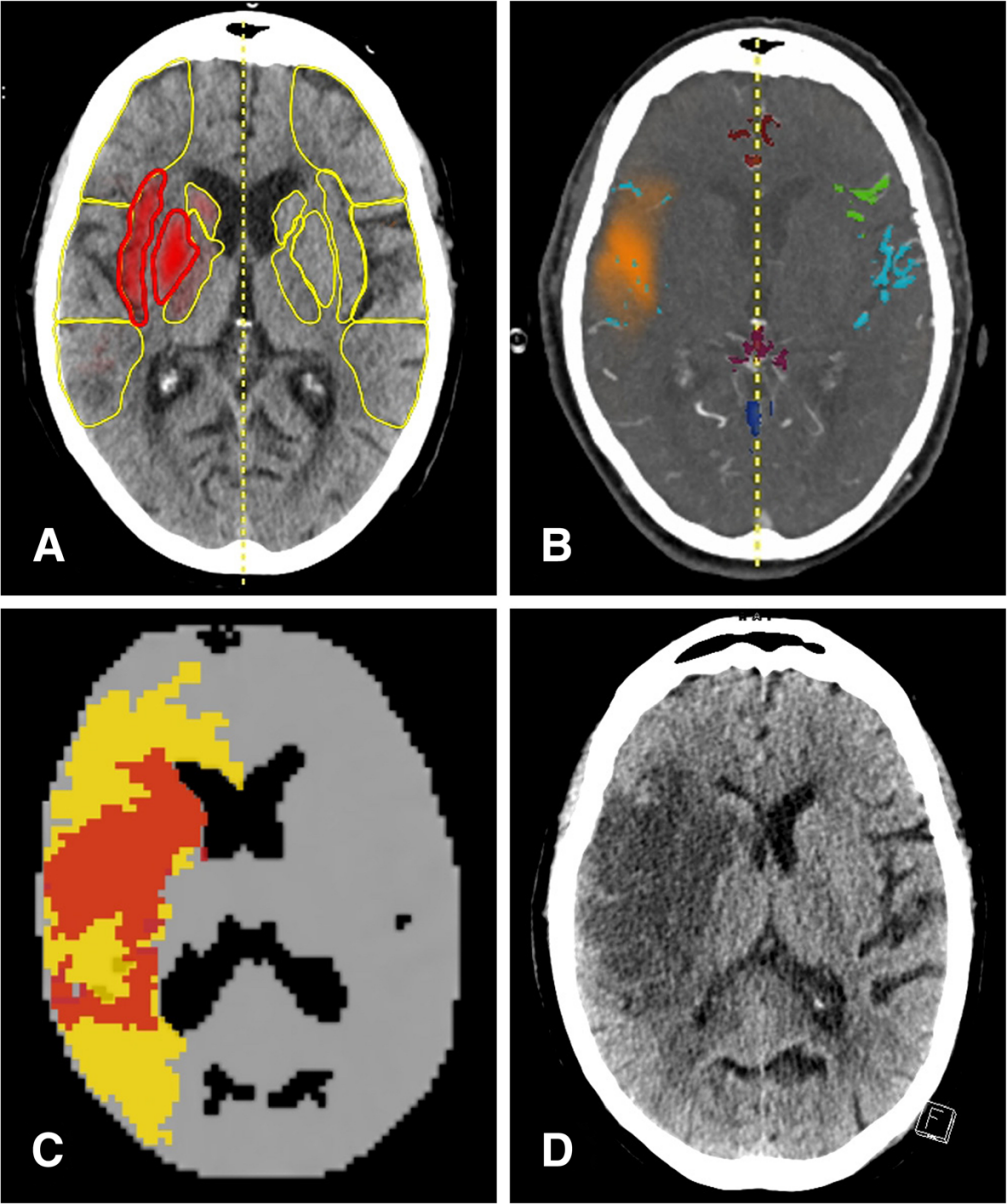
Acute LVO with insufficient collateral flow and extended infarction despite successful recanalization: An 84-year-old woman suffered from an acute hemiparesis (NIHSS 14) due to an M1 right-sided M1-occlusion. e-ASPECTS (a) was 8 due to early signs of infarction in the caudate head and lentiform nucleus, e-CTA collateral score (b) was 1 (21%), and there was a large area of hypoperfusion with an only moderate mismatch (c). Neurological deficit persisted (NIHSS 12) despite full recanalization (mTICI 3) within 5 h from symptom onset and follow up NCCT at 24 h (d) shows near complete infarction of the MCA territory. Note the difference of the arterial vessels (b, blue colour) compared to the opposite side as well as the reduced parenchymal contrast (b, orange cloud) on the affected side
